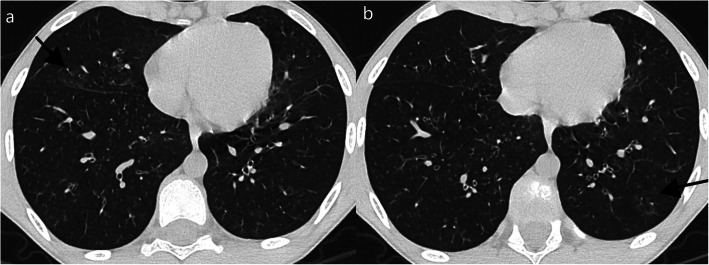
Indeterminate features in a 11-year-old with history of atopy that was coinfected with *Mycoplasma pneumoniae* and rhinovirus. Chest CT shows 2 very subtle nodular GGO, one not peripheral (a). Subtle centrilobular micronodules were seen in the lower lobes (b) suggesting small airway disease. These findings are not typical for COVID-19 disease. Diffuse bronchial wall thickening was also seen
